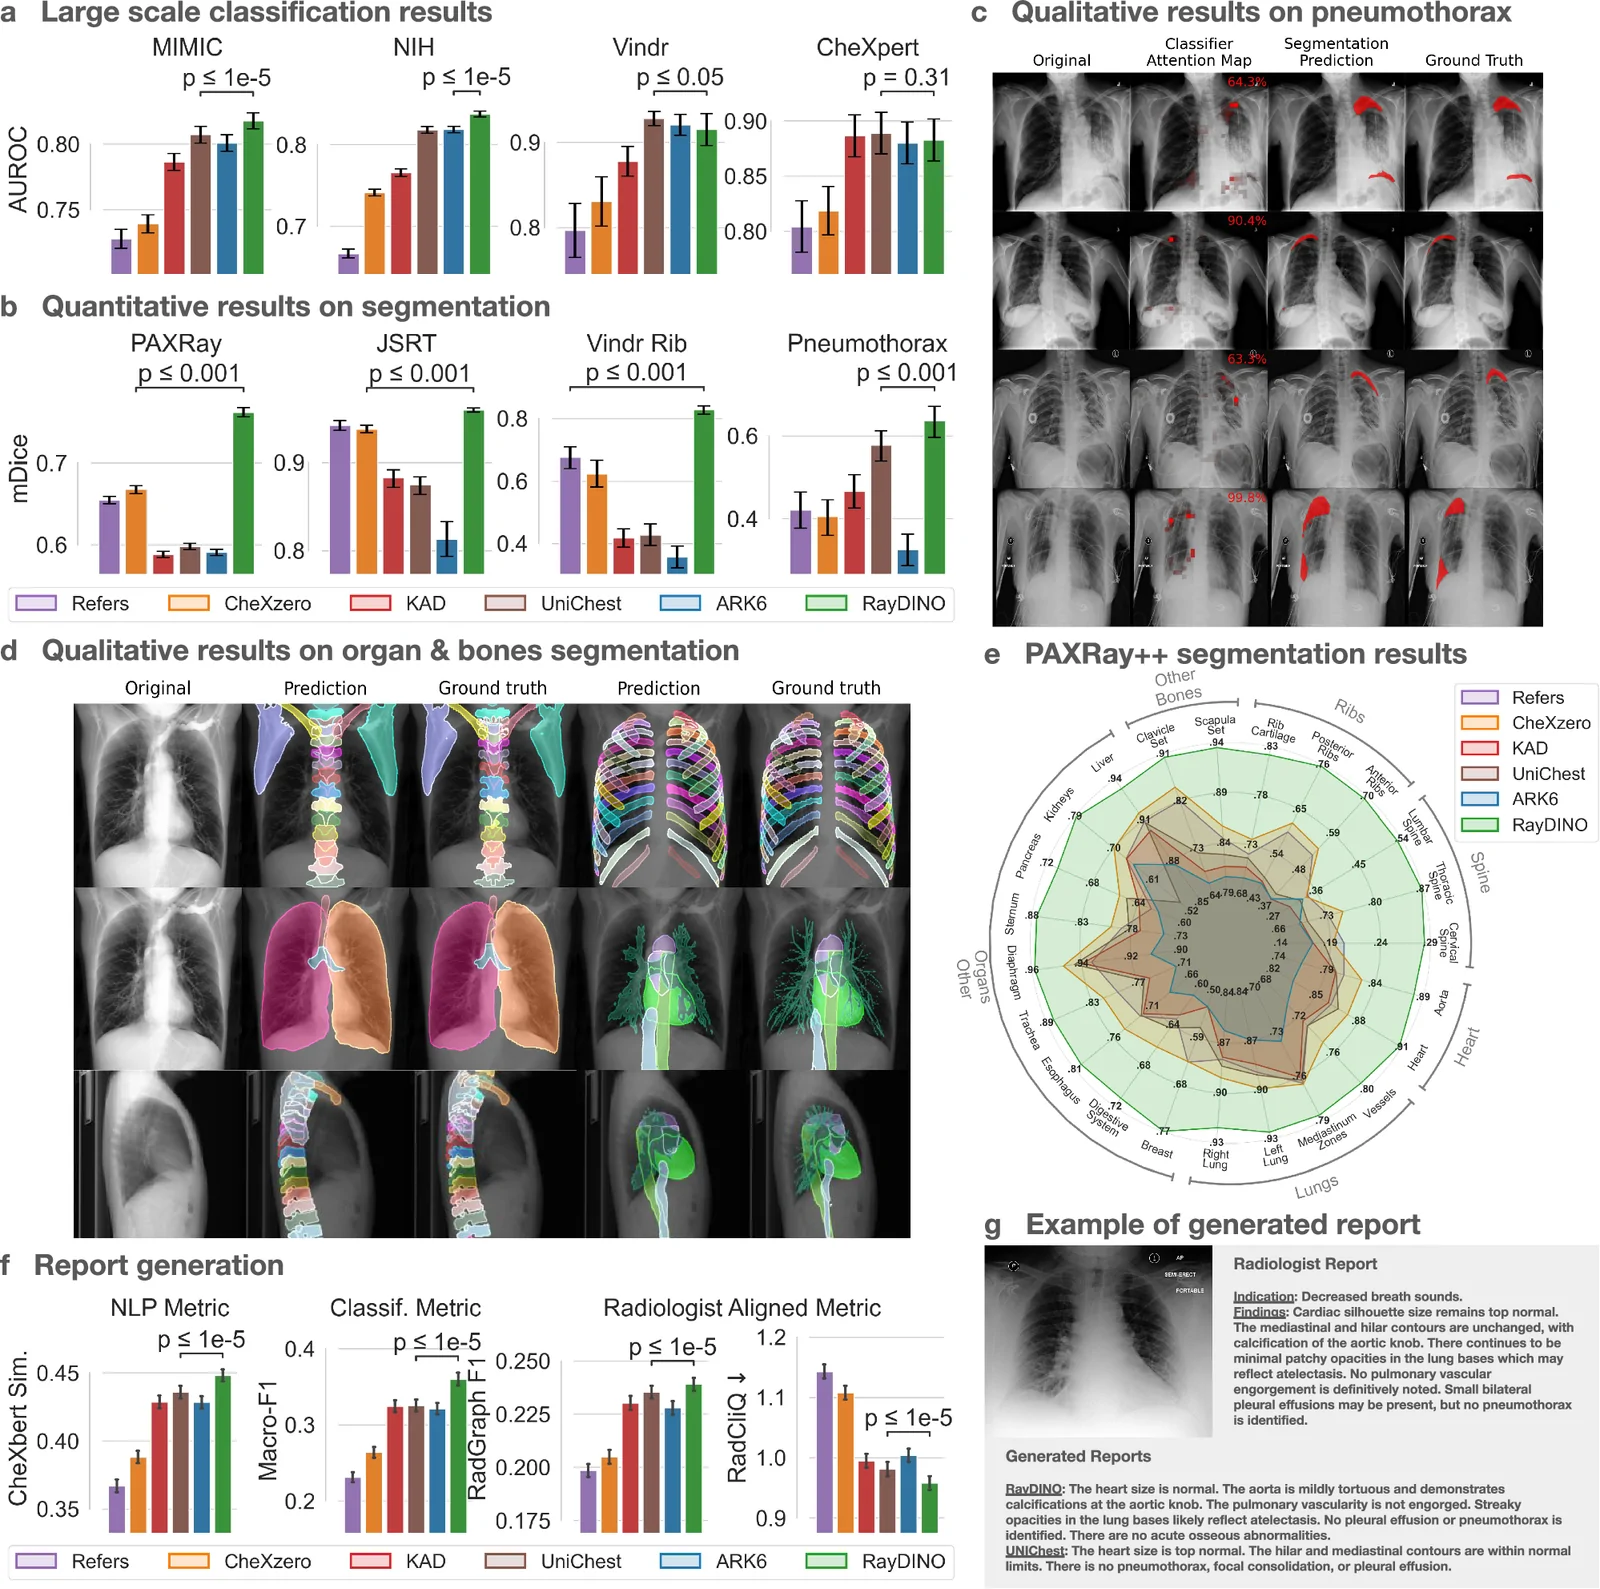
RayDINO’s holistic analysis of chest X-rays. a AUROC comparisons on 4 classification datasets from the USA and Vietnam including 38 different findings (MIMIC *n* = 5159 test images, NIH *n* = 25, 596, CheXpert *n* = 668, VinDr *n* = 3000). b mDice comparisons on 4 segmentation datasets from Japan, Vietnam, the USA, China and multiple other countries, including 157 categories (JSRT *n* = 50, VinDr Rib *n* = 49, PAXRay++ *n* = 1, 476, Pneumothorax *n* = 3, 205). c Qualitative visualisation of RayDINO’s prediction on four pneumothorax cases. 1^st^column: original image. 2^nd^column: interpretable attention maps showing where the classifier trained without pixel supervision is looking at. 3^rd^column segmentation prediction trained using pixel supervision. 4^th^column: radiologist ground-truth. d Qualitative results for organ and bone segmentation on the PAXRay++ dataset. e mDice segmentation comparisons macro-averaged per organs and bones (*n* = 1, 476 test images). f Report generation comparison including natural language processing metric, classification-based metric and radiologist-aligned metrics (*n* = 3, 822 test reports). g A chest X-ray along with a radiologist report and two generated reports by RayDINO and UNIChest. In a, b, e and f, data are presented as mean values ±95% confidence intervals obtained via bootstrapping (1000 resamples, 2.5th–97.5th percentiles). Statistical significance was assessed using a two-sided DeLong test for AUROC comparisons and a two-sided paired permutation test (1000 bootstraps) for all other metrics; no adjustments were made for multiple comparisons. Exact p-values are reported in the main text.
